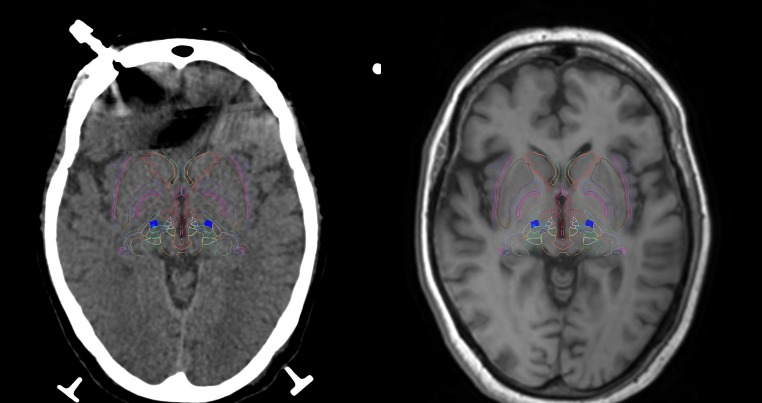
Vectorial depictions of the thalamus and basal ganglia superimposed on para-transverse images Vectorial depictions of the thalamus and basal ganglia (colored lines) are superimposed on para-transverse computed tomography (CT) (left) and magnetic resonance (MR) (right) images. These images are reconstructed from stereotactic CT images and geometrically aligned, non-stereotactic MR images, respectively, in the coordinate system of a vectorial model of the thalamus and basal ganglia that is based on stereotactic atlases [9-10]. The coordinate system of the vectorial model is defined by the anterior commissure (AC), posterior commissure (PC), and a point on the mid-sagittal plane. The model is scaled automatically to match the AC-PC distance prior to superimposition on the para-transverse images and may be rescaled manually thereafter. In each para-transverse image, the target point is depicted by a cyan circle.
